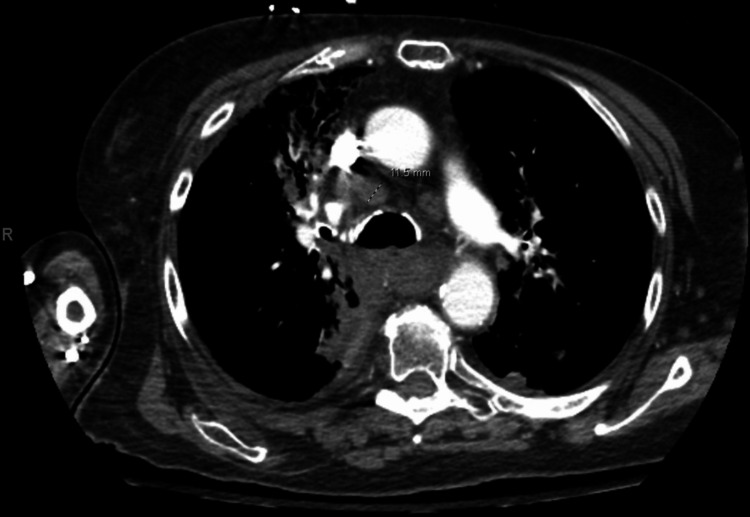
CT scan showing precarnial/right paratracheal lymph node, largest measuring 1.2 cm and showing esophageal dilation with layering debris proximal to the level of subcarinal lymph node compression. Airspace opacities noted.
